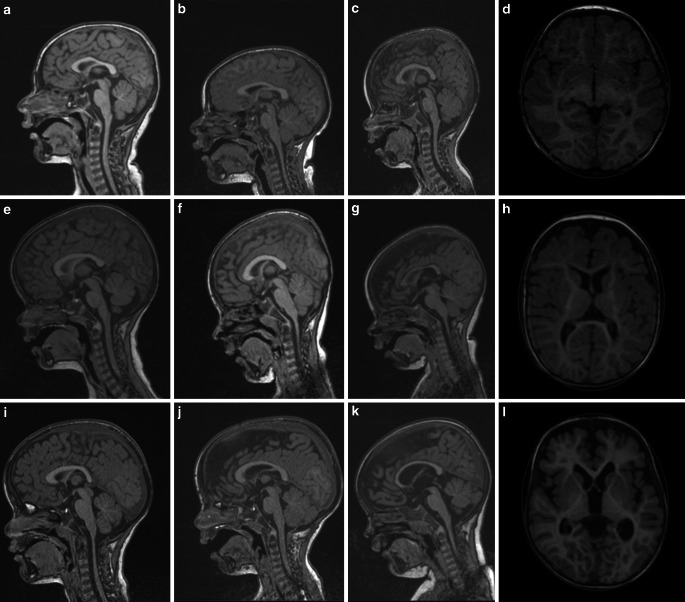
Midsagittal reformats of 3D T1-weighted MPRAGE (Magnetization Prepared – RApid Gradient Echo) sequences with FOV height of 25 cm. As children have different ages (10 months to 3 years) and FOVs are adapted to the head sizes as depicted, it is difficult to assess which children are microcephalic, have a normal head size or are macrocephalic. Microcephaly: a male, 3 years, 47 cm HC (0.7 cm < 3rd percentile), b male, 4 years, 47 cm HC (1.2 cm below the 3rd percentile), c male, 15 months, 43 cm HC (1 cm < 3rd percentile). Normal HC: e female, 3 $$\frac{10}{12}$$ years, 51.5 cm HC (92nd percentile), f male, 3 $$\frac{4}{12}$$ years, 50 cm HC (24th percentile), g male, 10 months, 45 cm HC (33rd percentile). Macrocephaly: i male, 3 $$\frac{10}{12}$$ years, 53 cm HC (0.5 cm > 97th percentile), j male, 3 $$\frac{4}{12}$$ years, 56.2 cm HC (2.8 cm > 99th percentile), k female, 24 months, 51 cm HC (0.5 cm > 97th percentile). By measuring the head circumference, which is exemplary shown in the right-hand column, a child’s head size can be easily classified: d (case c) clinical HC 43 cm, MRI-based HC 435 mm, h (case g) clinical HC 45 cm, MRI-based HC 440 mm, l (case k) clinical HC 51 cm, MRI-based HC 505 mm
